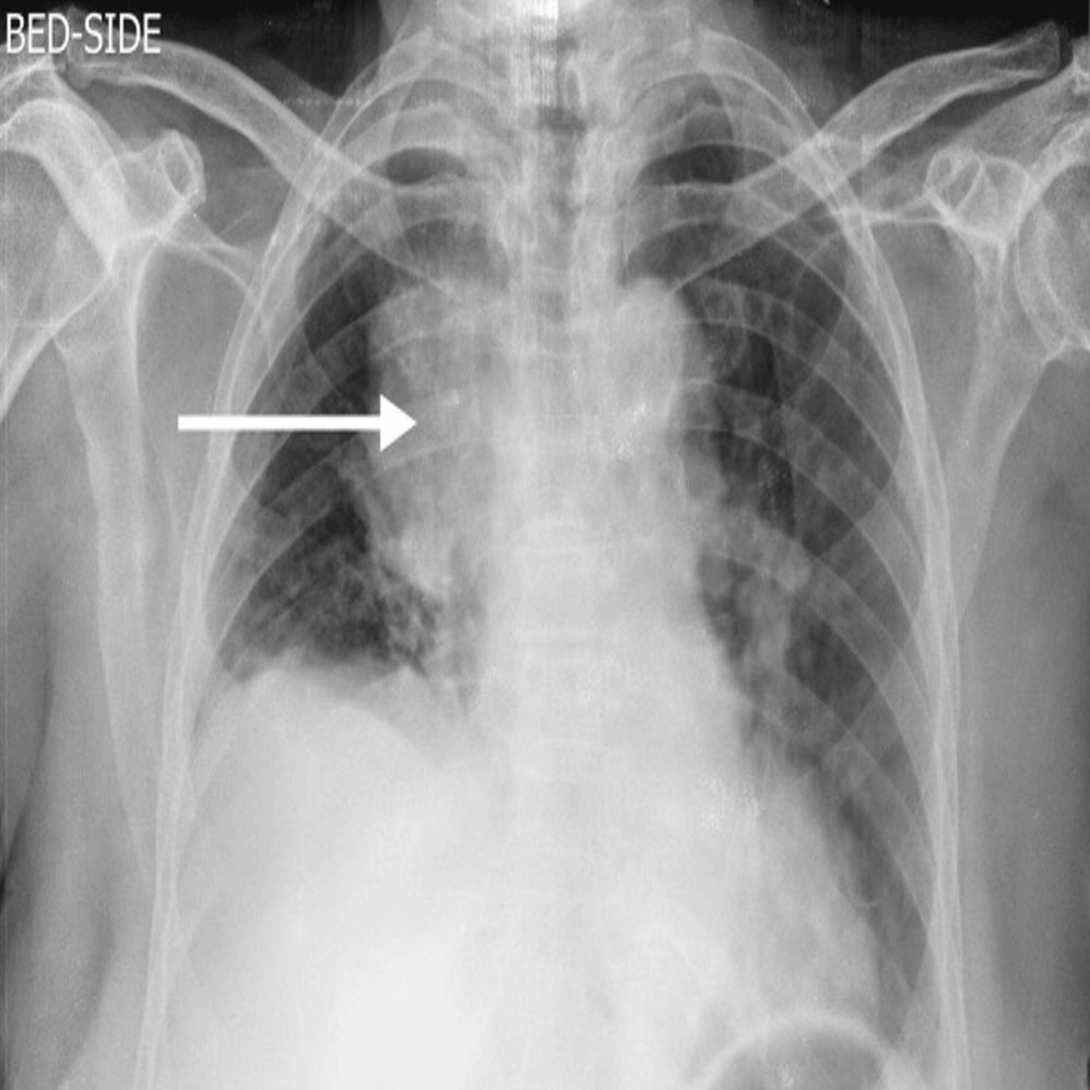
Chest X-ray showing right-sided homogeneous opacity, silhouetting right heart border
